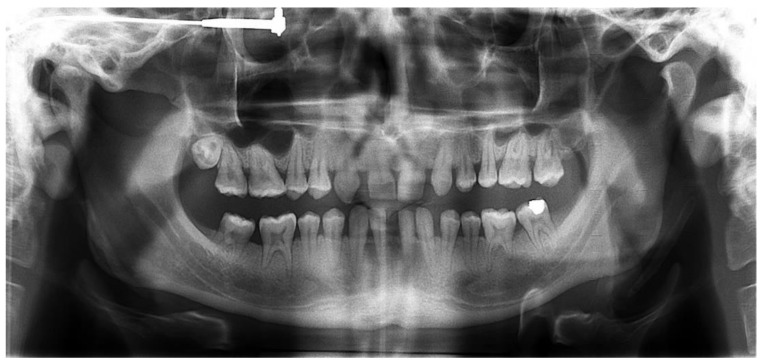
Orthopantomogram of the patient’s initial dental condition in February 2021.
